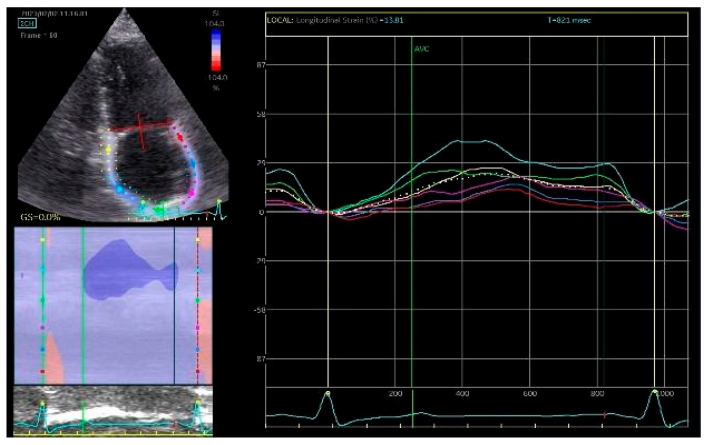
Assessment of LA strain in apical 2-chamber section.
